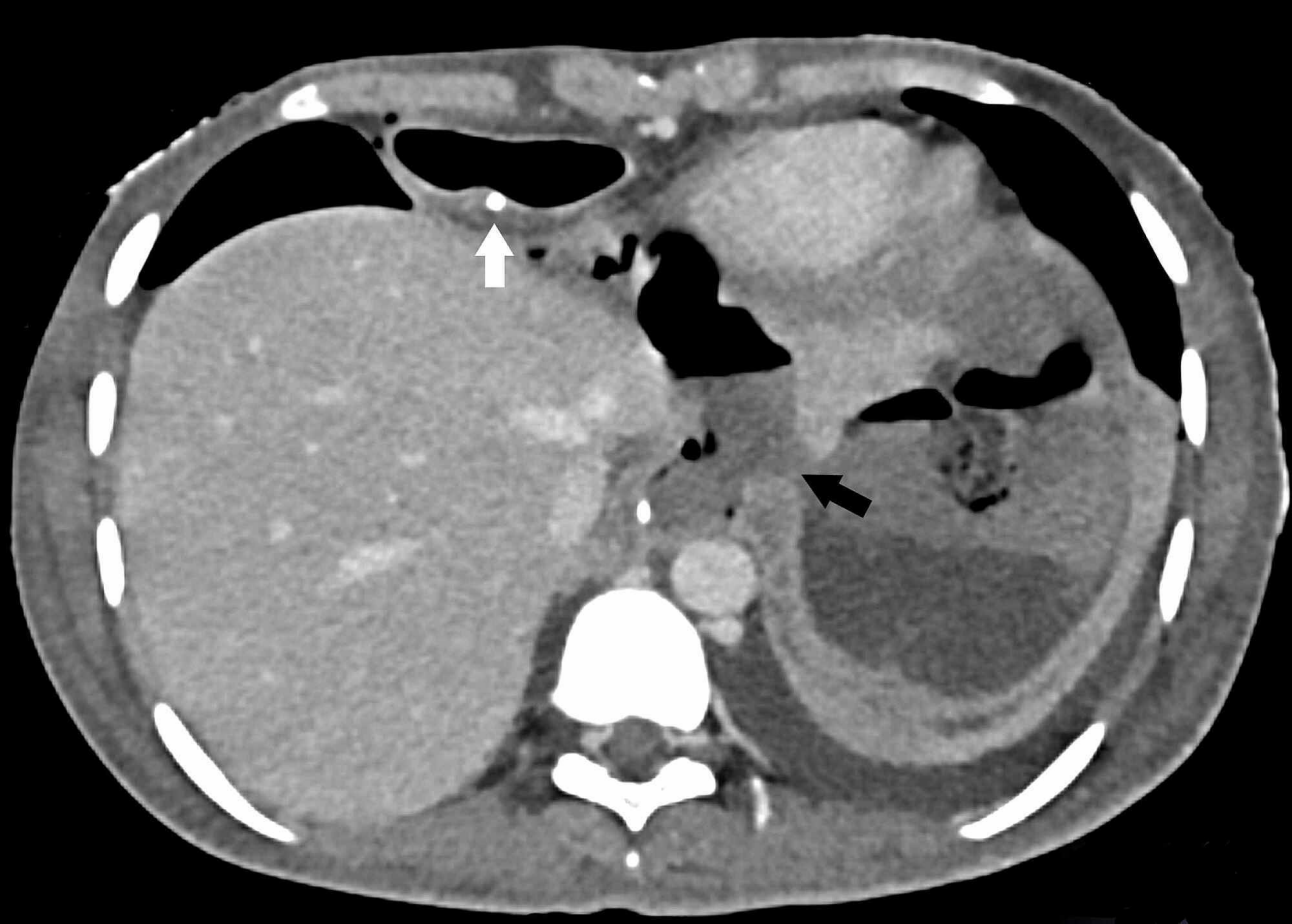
Axial view of abdominal computed tomography with contrast showing the site of leak from the intraabdominal esophageal staple line (black arrow) and the intrabdominal part of the gastric pull-up with intact staple line (white arrow).
